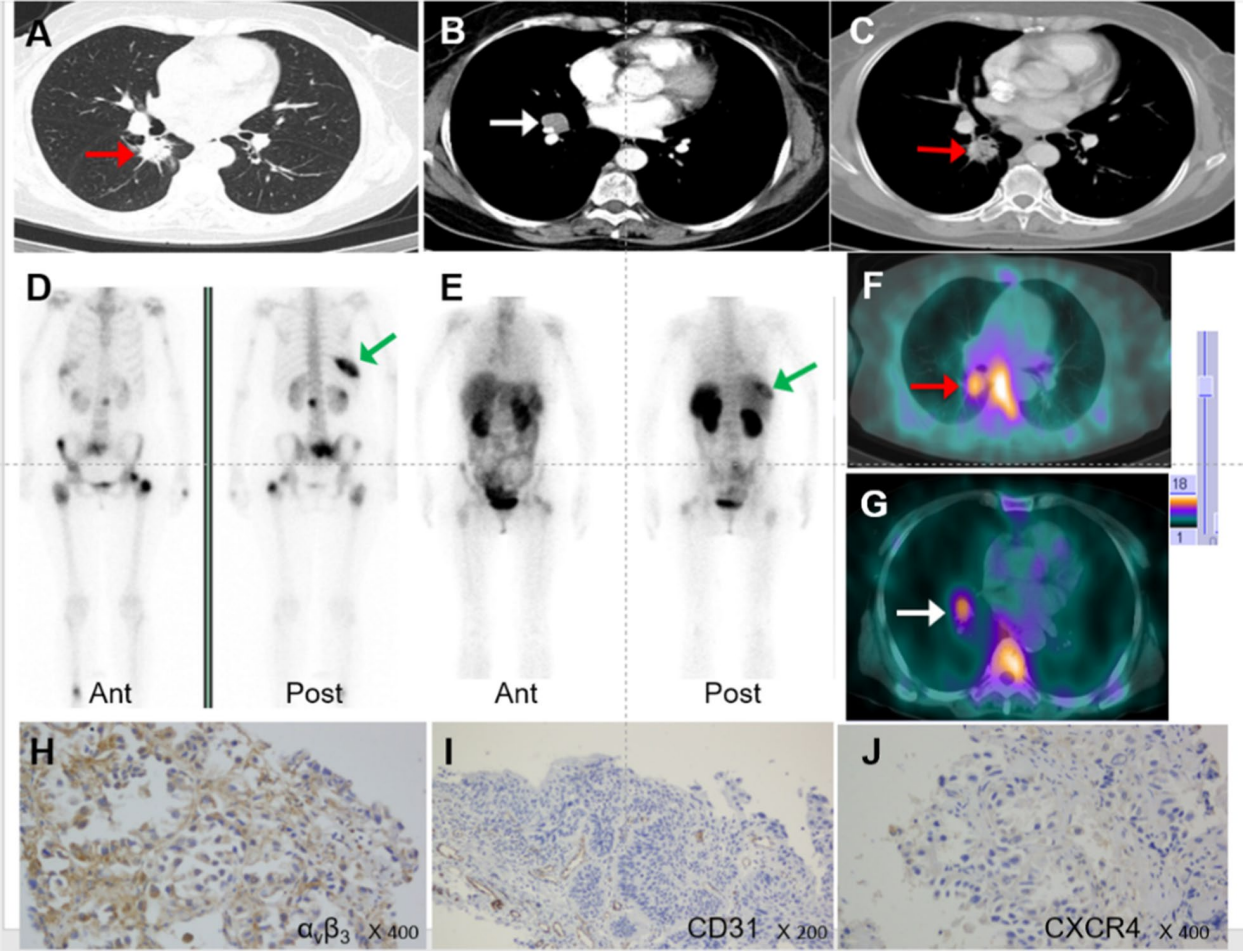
[^99m^Tc]Tc-Galacto-RGD_2_ imaging showed RGD-avid uptake in the primary tumour, lymphadenopathy, and remote metastases in a patient with suspected multiple myeloma. The final diagnosis was lung adenocarcinoma confirmed by bronchoscopic biopsy. a Primary lesion presented in the lung window. b, c Enhanced CT showed primary tumour and lymphadenopathy in the right hilum. d, e Bone scan showed lytic lesion in the right rib and sclerotic lesions in the pelvis. f, g
